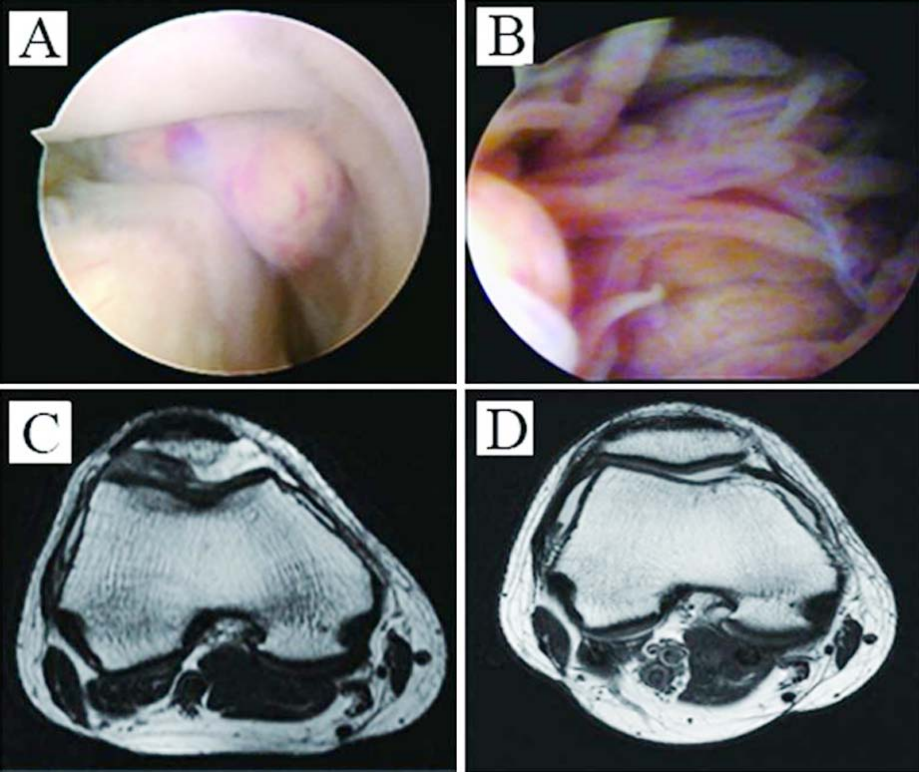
Intra-operative arthroscopic diagnosis for (A) localised type and (B) diffuse type; (C) pre-operative MRI examination; (D) MRI examination of the same patient at 5 years after the operation.
